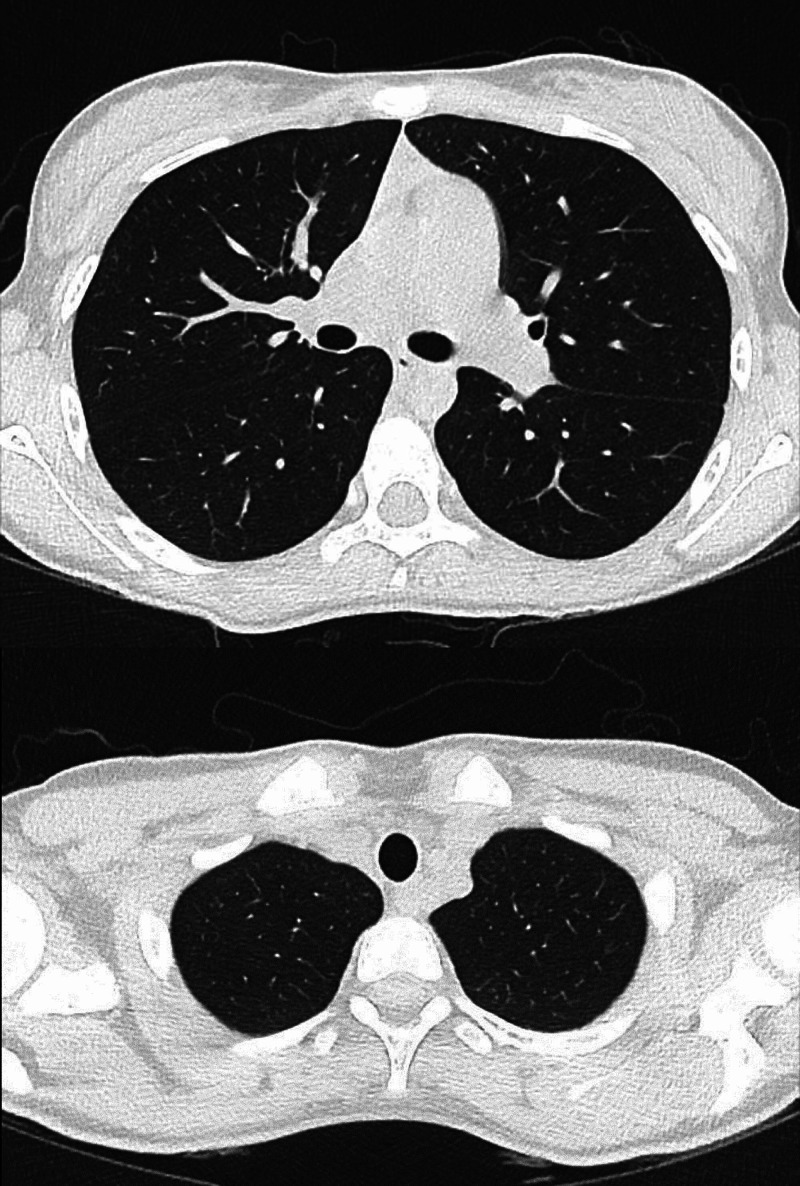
CT Thorax showing resolution of the pneumothorax and no evidence of blebs or bullae
